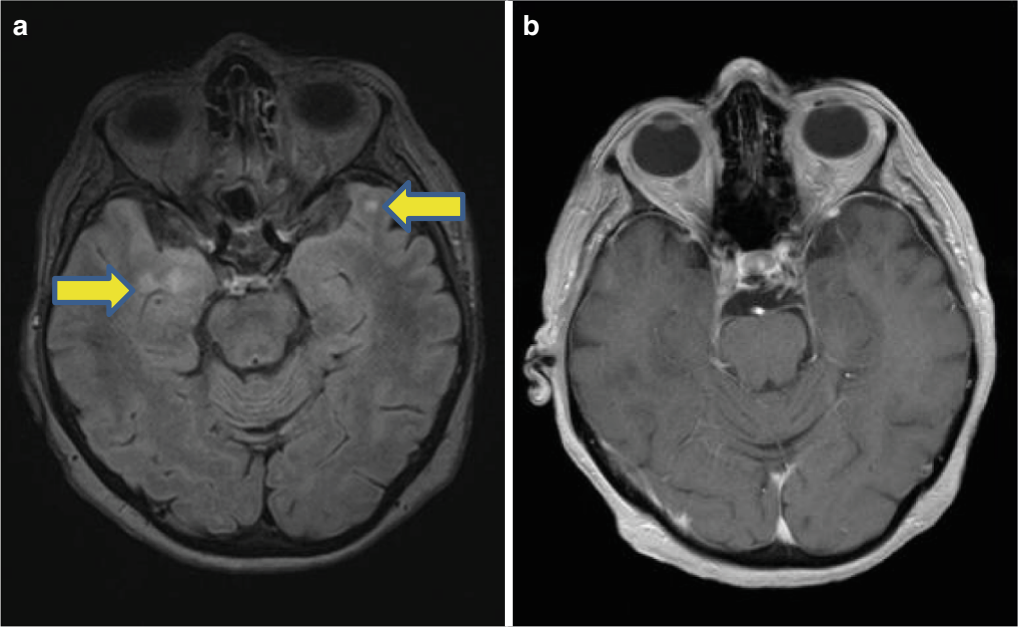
a Cerebral MRI T2-weighted (fluid-attenuated inversion recovery); *yellow arrows* indicate bitemporal hyperintense signals. b Cerebral MRI T1-weighted + gadolinium: no contrast enhancement
